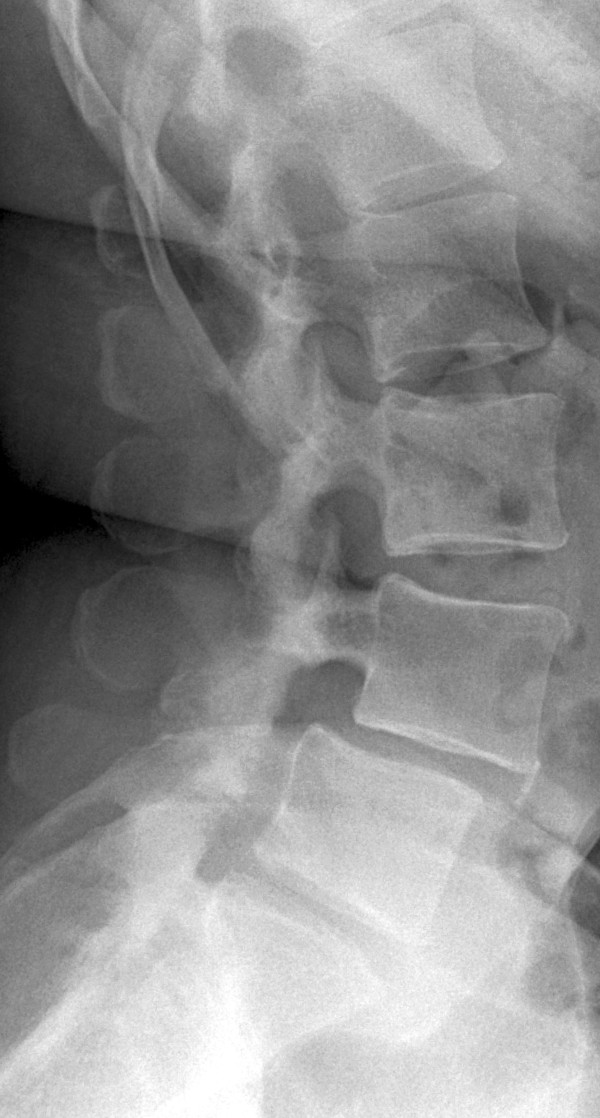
X-ray taken before the patient’s first surgery.
